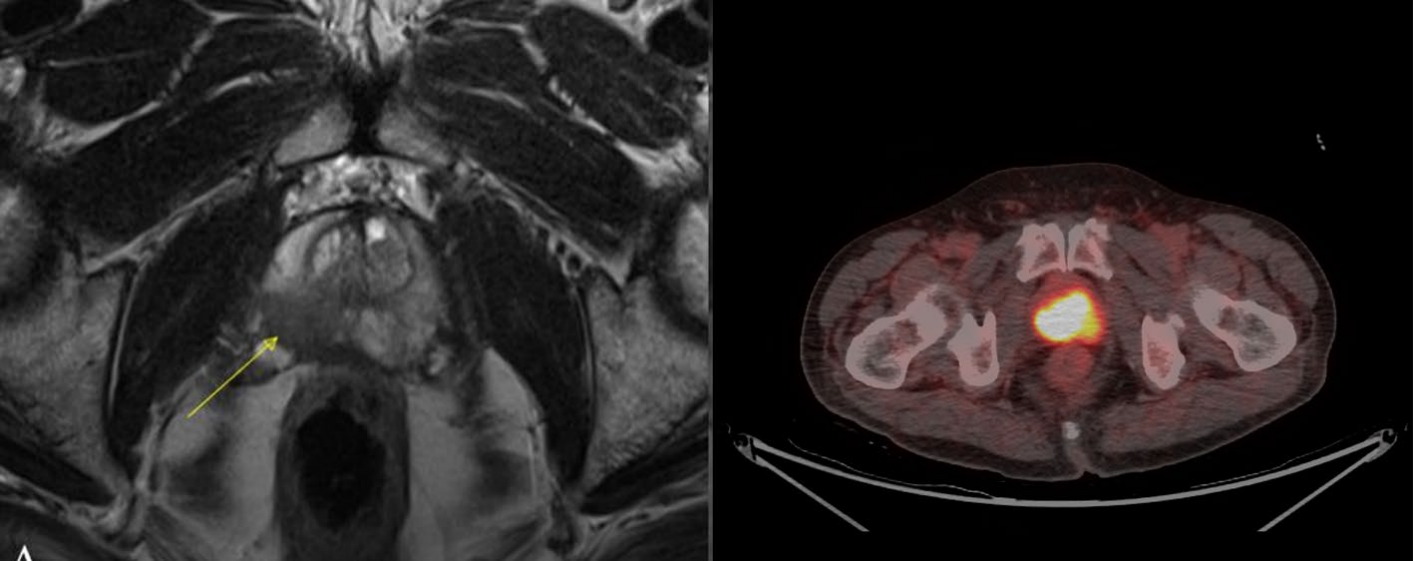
Patient with mpMRI showing PIRADS 5 lesion with intense tracer uptake in the heterogeneously enhancing mass involving the entire prostate gland from base to apex (SUVmax 35.1). Biopsy revealed adenocarcinoma Gleason grade 3 + 4
